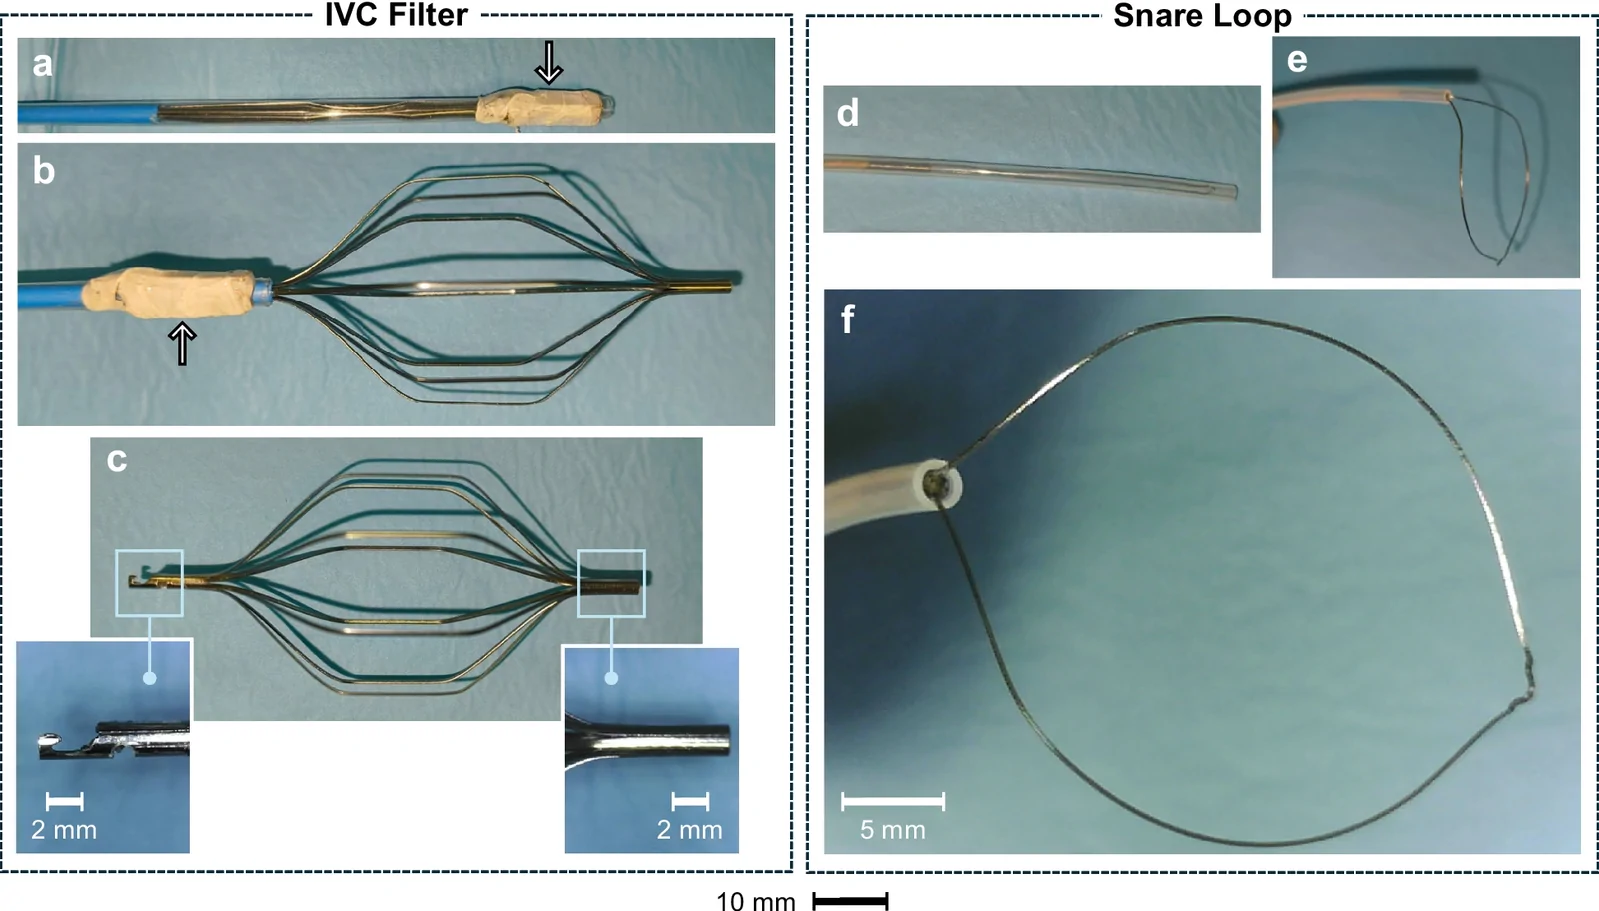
Inferior vena cava (IVC) filter and snare loop (SNL) designed for MRI-guided interventions. For catheter-guided delivery of the IVC filter, a custom-built guiding catheter equipped with a semi-active MR-resonant marker was used, whereas SNL–assisted retrieval was performed using a catheter with passive iron-oxide markers. a IVC filter positioned inside the semi-actively marked delivery catheter; the MR-resonant saddle coil marker is indicated by the white arrow. b IVC filter deployed from the delivery system; MR-resonant saddle coil marker indicated by the white arrow. c IVC filter with integrated resonant circuit design. The left inset shows a magnified view of the retrieval hook, while the right inset depicts the cranial-facing end of the IVC filter. d MR-resonant SNL integrated into a dedicated catheter-based delivery system with passively marked MR Conditional shaft. For retrieval, the system was used in combination with an outer retrieval catheter. e SNL deployed from the catheter. f Magnified view of the SNL
